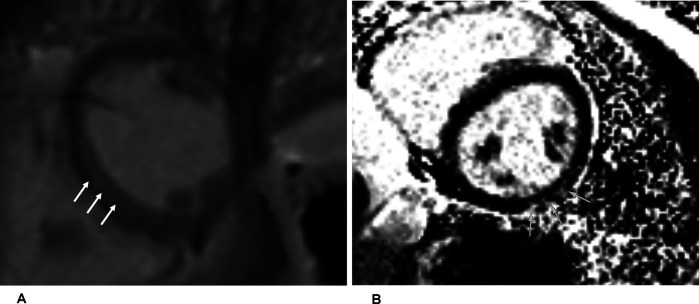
Magnetic resonance imaging of the heart (short-axis views). LGE is displayed in the epicardial midventricular (A) and patchy inferolateral (B) areas. The arrows refer to the LGE.
